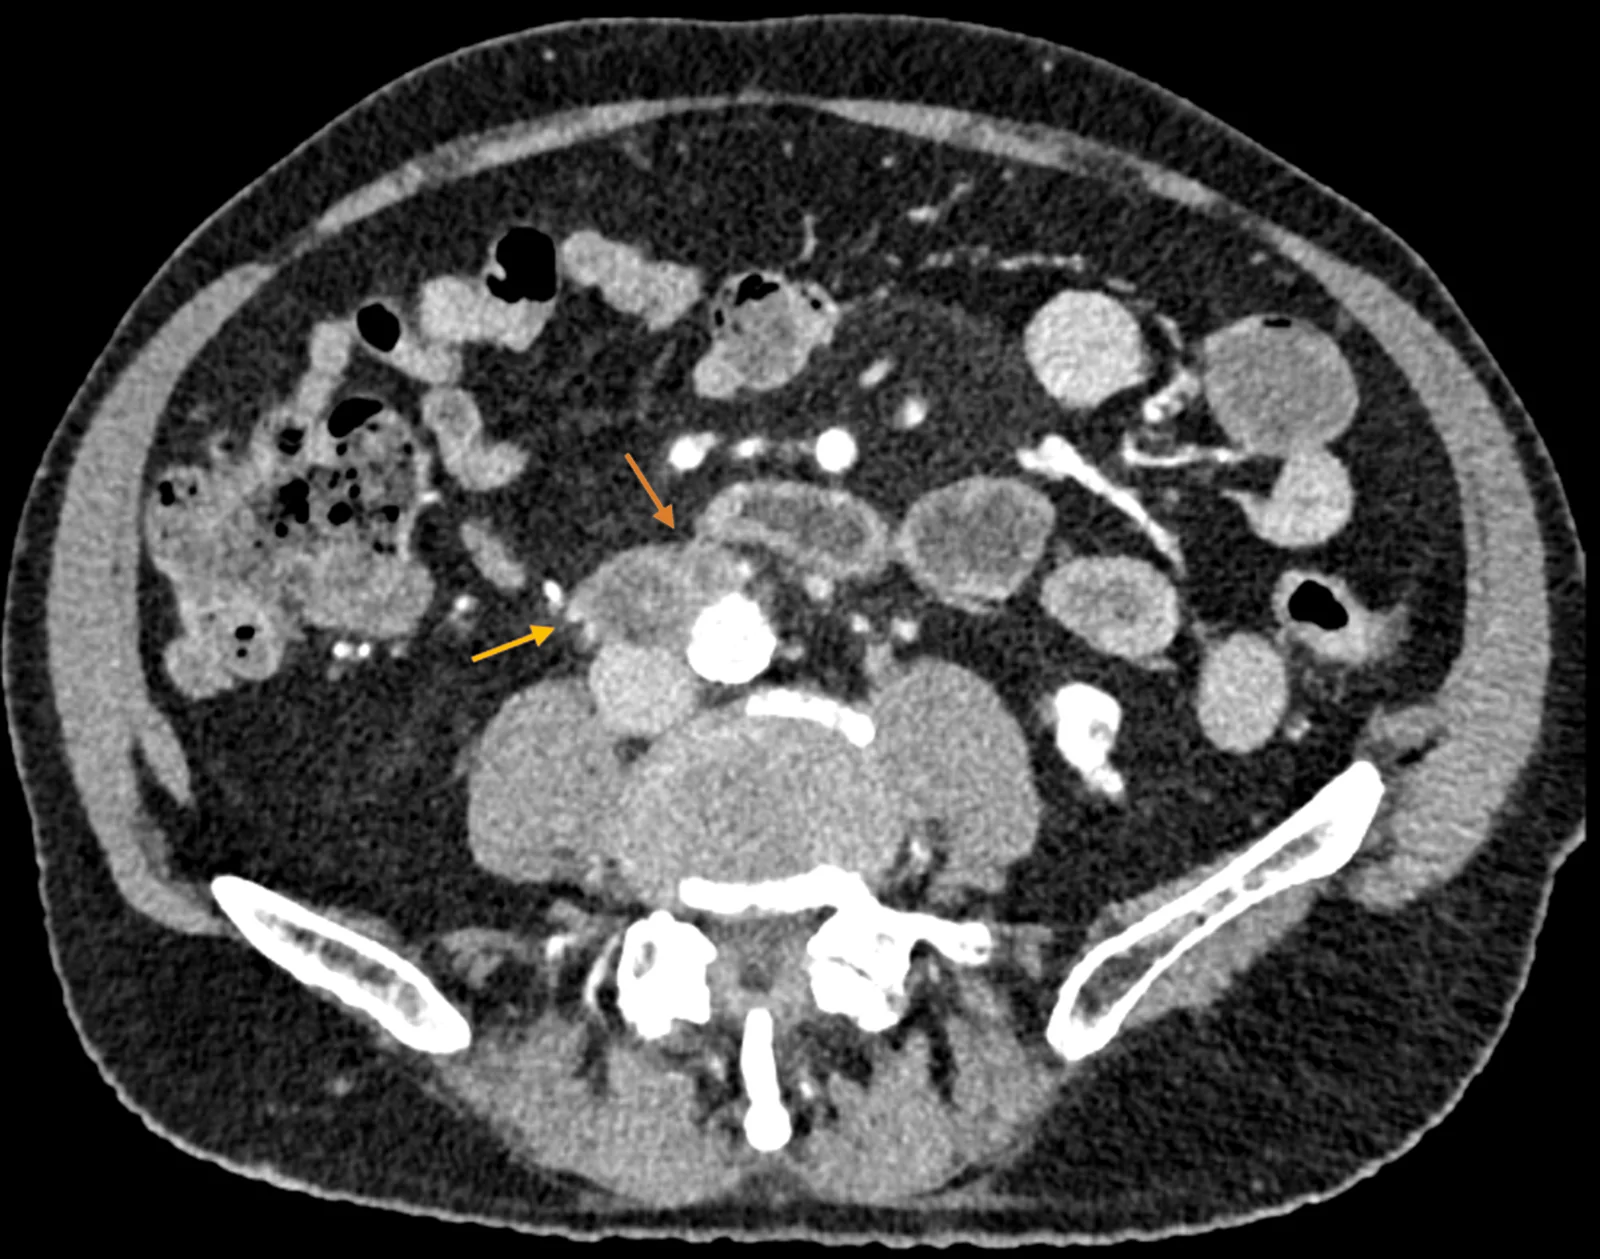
Contrast-enhanced axial CT image demonstrating progressive enlargement of the pre-aortic (orange arrow) and precaval (yellow arrow) lymph nodes.
